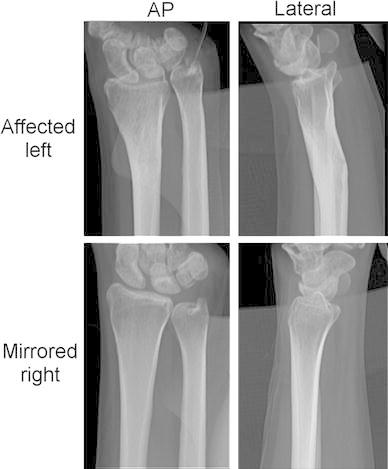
Anteroposterior and lateral radiographs of the affected wrist (*top row*) revealing a malunion of the radius and of the mirrored healthy wrist (*bottom row*)
